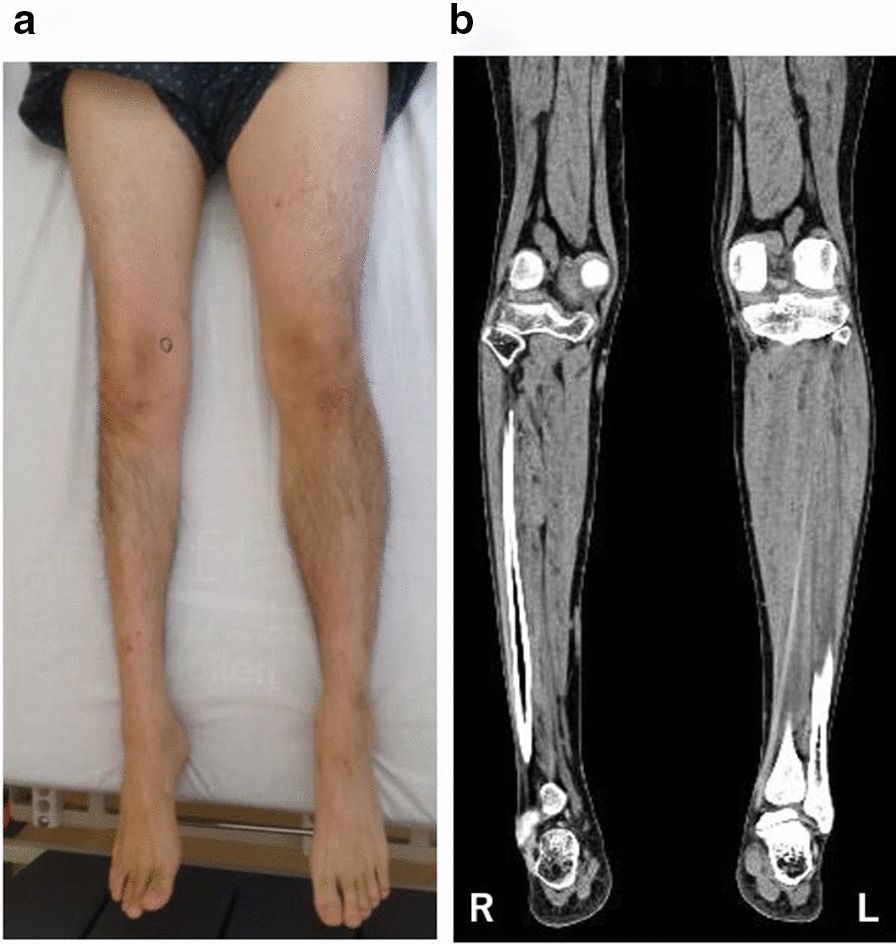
Muscular atrophy of the patient’s right leg. a Photograph. b Computed tomography (CT)
